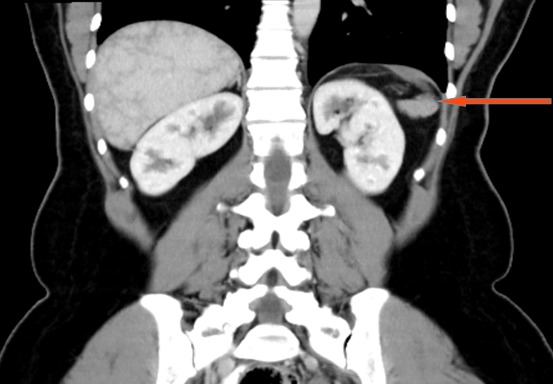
Post-surgery coronal abdomen CT: shows several splenules, no spleen (red arrow)
